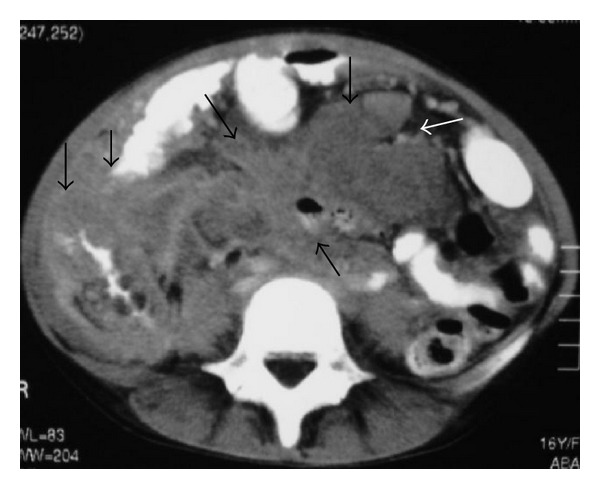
NHL. Axial CT shows masses involving small bowel loops and ascending colon (arrows).
